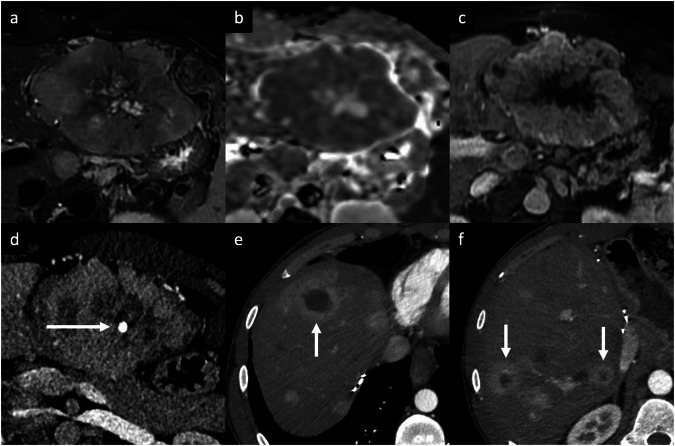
FLC in a 29-year-old woman. a Axial T2-weighted MR sequences show a mildly hyperintense heterogeneous mass in the left liver lobe, containing a T2-hypointense central scar, (b) with a targetoid appearance on an ADC map. c Extracellular contrast-enhanced AP image shows heterogeneous enhancement within the mass. d Axial contrast-enhanced AP CT demonstrates heterogeneous enhancement with typical central calcification (arrow). e, f Contrast-enhanced CT imaging performed after two years of a surgical tumor resection shows multiple tumor recurrences on the right hepatic lobe with rim APHE (arrows)
